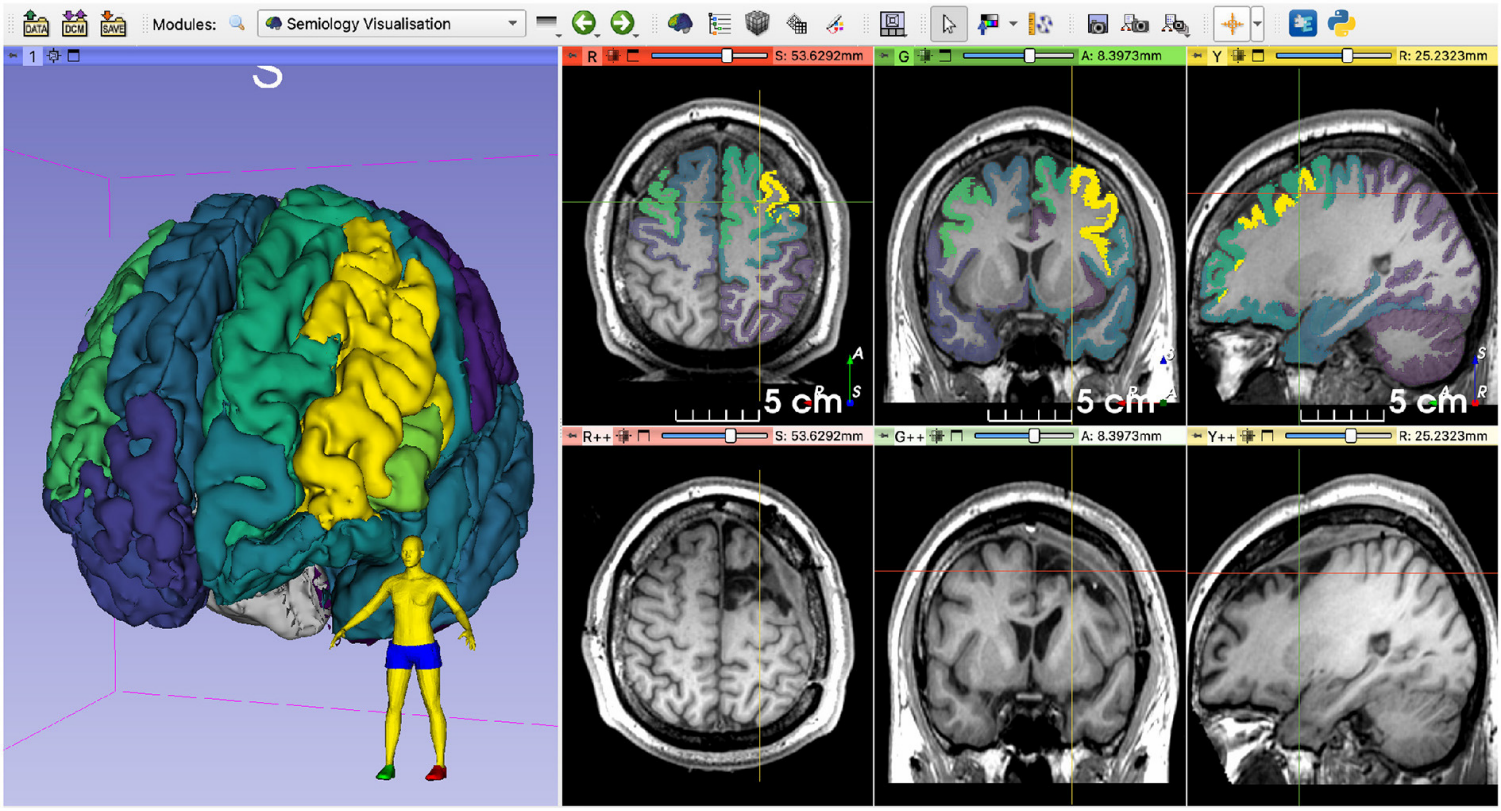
Qualitative comparison of the EZ probability map and the postoperative MRI. Note the overlap between the highlighted structures and the resection cavity. The images were rigidly registered, and the crosshairs are centered on approximately the same region. The patient became seizure-free after resective surgery.
